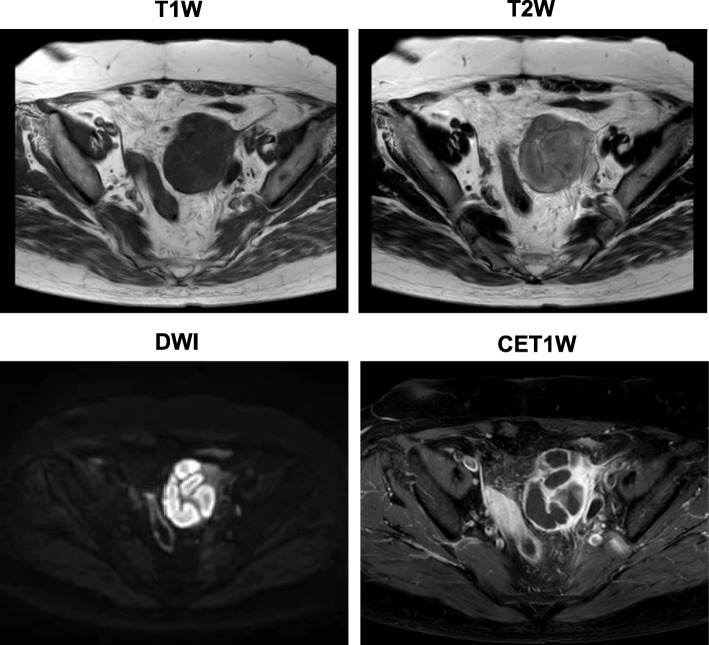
Magnetic resonance imaging axial T1-weighted images through the pelvis showed a markedly dilated fallopian tube posterior to the left ovary. Axial T2-weighted images showed a slightly higher intensity. Axial diffusion-weighted images and contrast-enhanced T1-weighted images showed a high intensity lesion at the same place. *CET1W* contrast-enhanced T1-weighted image, *DWI* diffusion-weighted image, *T1W* T1-weighted image, *T2W* T2-weighted image
